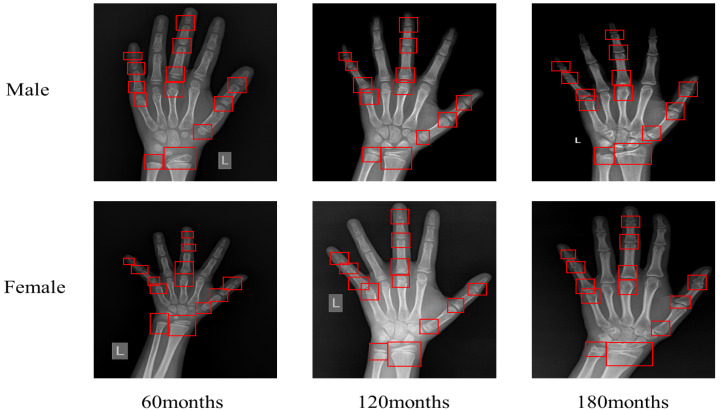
Left hand X-ray images of male and female children at different ages. The red boxes are the ROIs.
